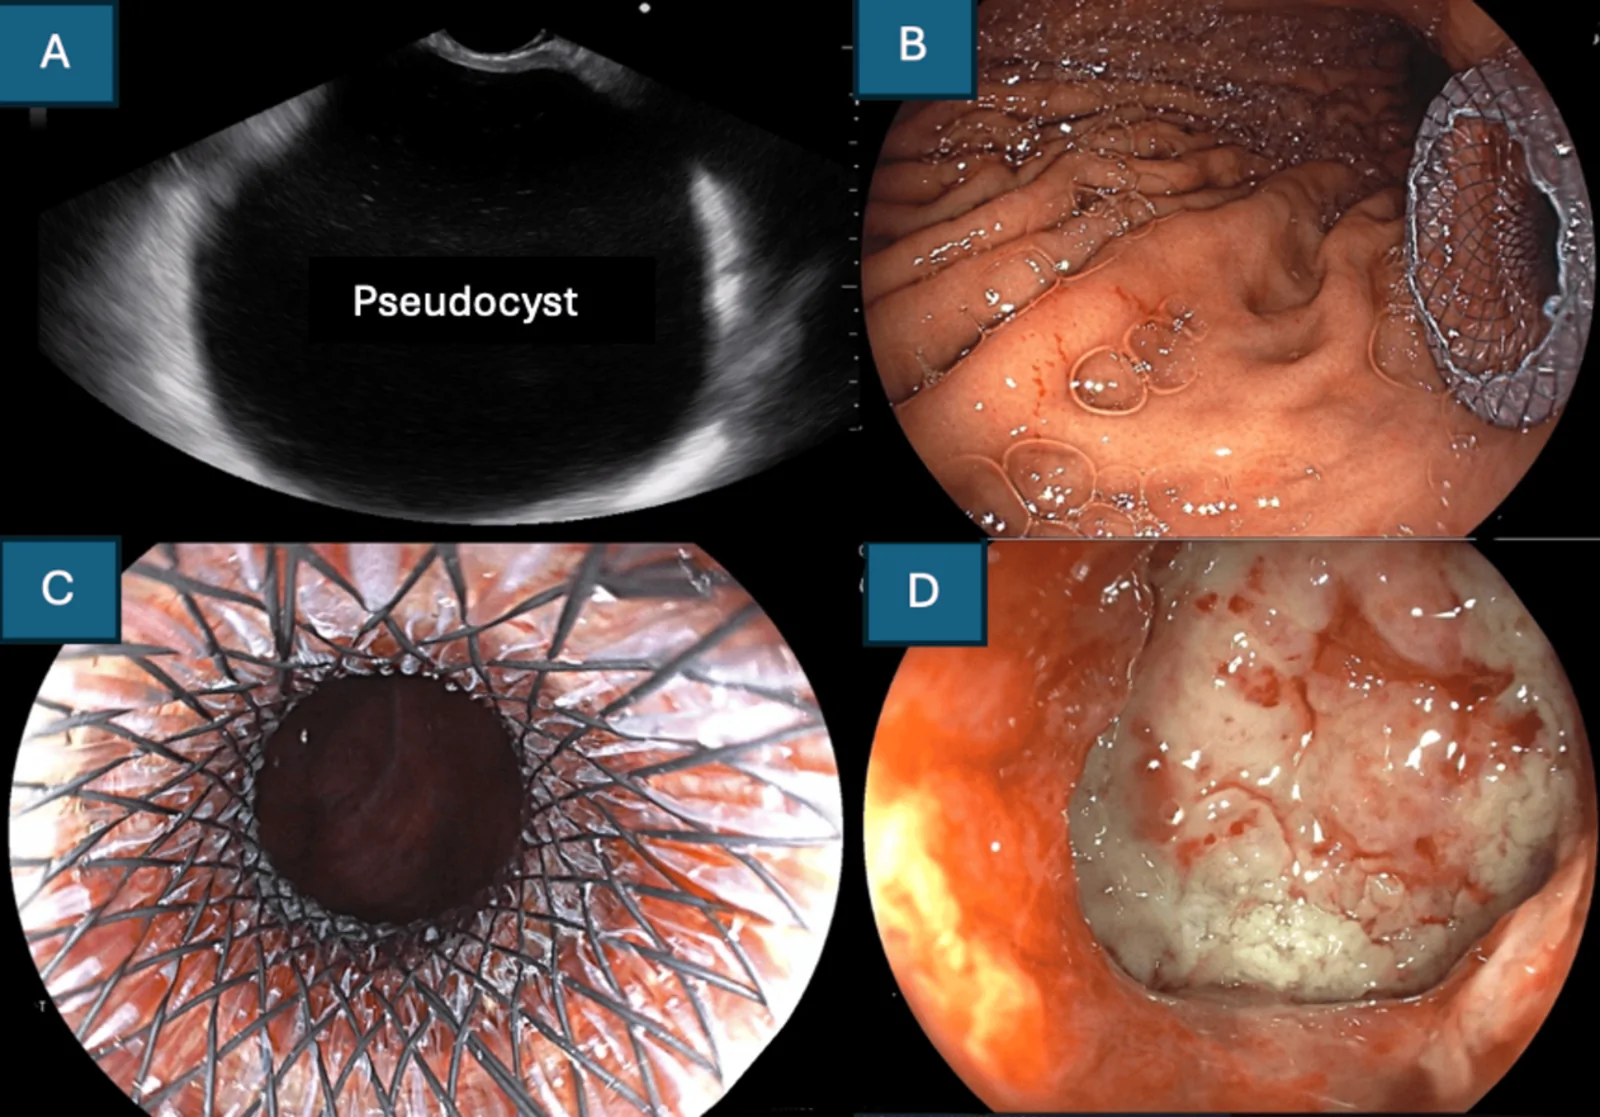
Endoscopic ultrasound (EUS) view of a 10-cm pancreatic pseudocyst. (A) EUS view of a 10-cm pancreatic pseudocyst. (B) Drainage using a lumen-apposing metal stent (LAMS). (C) Endoscopic view of the interior of the cystic cavity. (D) Resolution of the cystic cavity four weeks after drainage.
